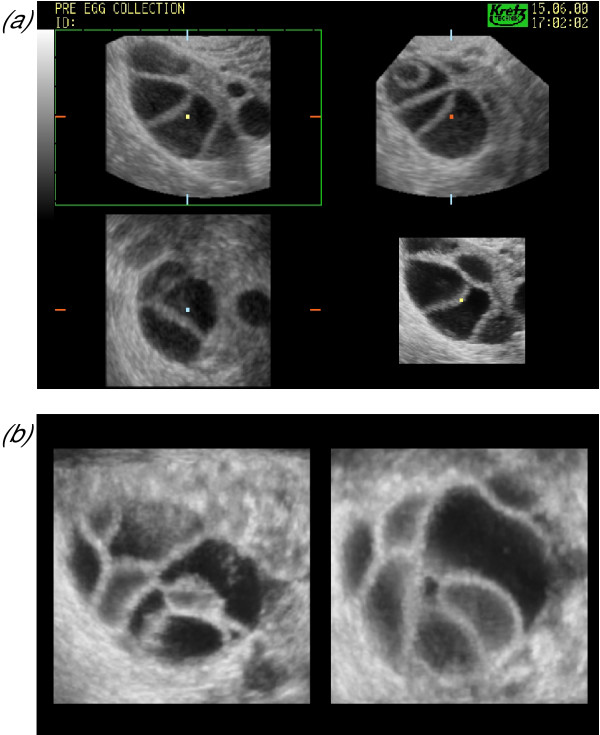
Controlled ovarian stimulation*. Three-dimensional sonography may be used to facilitate standard measurement of follicular diameter (a). During the latter stages of ovarian stimulation the ovaries may contain many follicles, which become progressively harder to measure reliably between observers, as there are no landmarks to aid orientation. Off-line analysis of stored three-dimensional datasets reduces the time spent with the patient and may potentially improve the number of mature oocytes retrieved by identifying the most appropriate time for oocyte collection. Evidence also suggests that three-dimensional rendering allows demonstration of the cumulus oophorus complex in follicles containing a mature oocyte (b).
